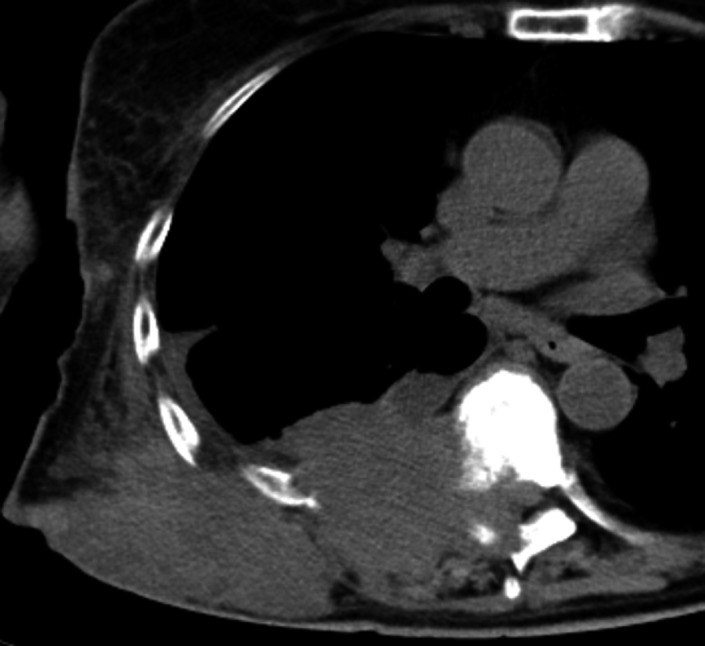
Thoracic CT image of patient with lower extremities paralysis. The tumor in the right latissimus dorsi invaded into the T7 vertebral body and spinal canal and has caused paralysis of lower extremities
